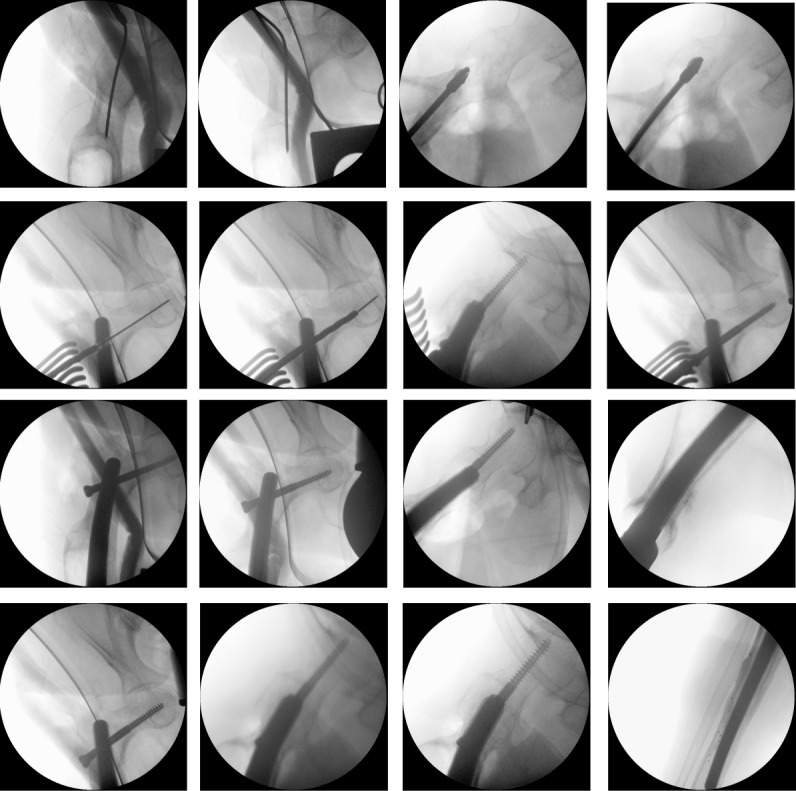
Intraoperative radiographs
